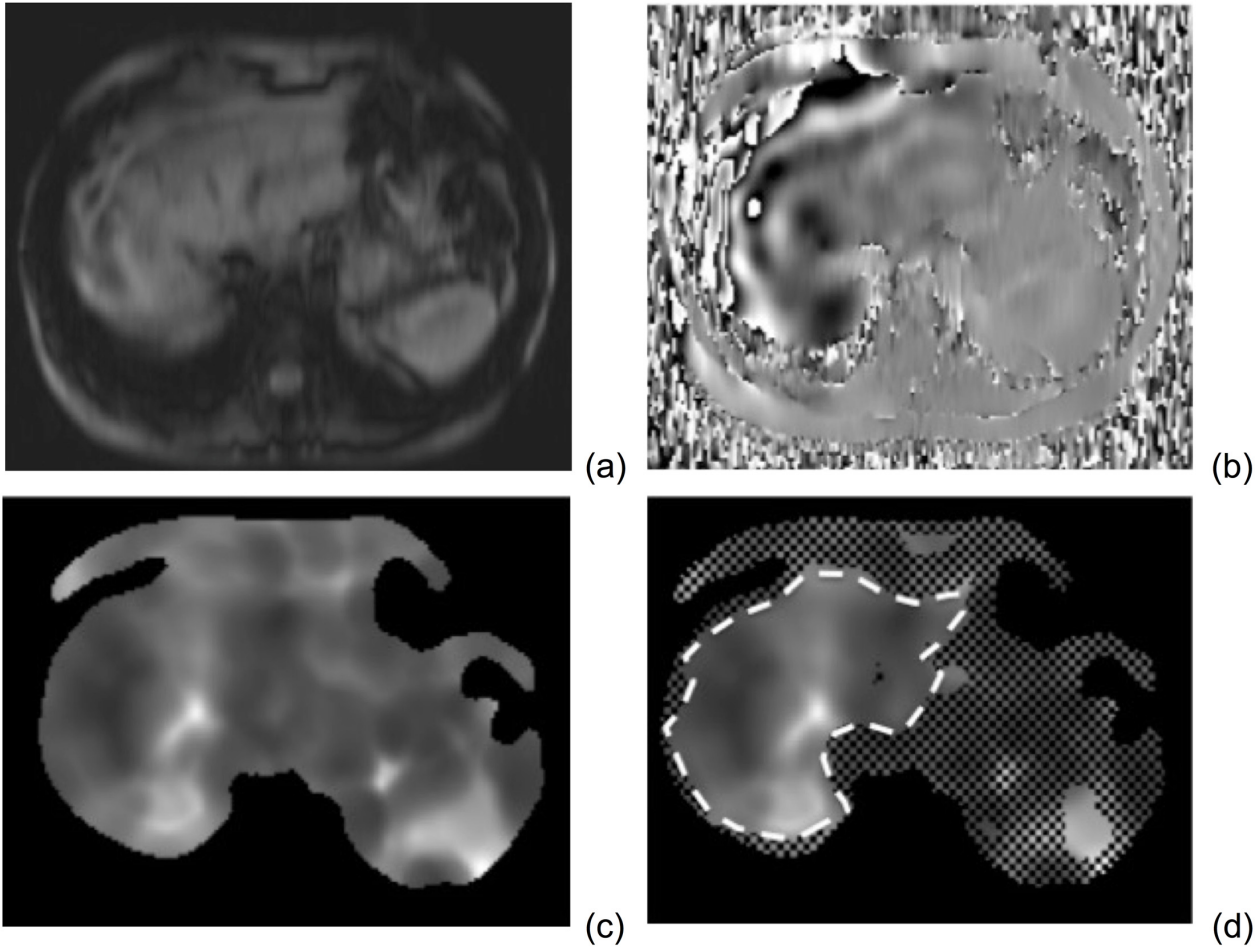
MRE data in a 46-year-old female with hepatitis B. (a) magnitude image,.(b) wave image, (c) stiffness map, and (d) confidence map. All images are at the same level. The dotted lines on the confidence maps represent the liver outlines of the corresponding patient’s MR images. The overall mean stiffness value on a total of 5 MRE images was 2.34 kPa. Liver biopsy confirmed fibrosis stage F1.
